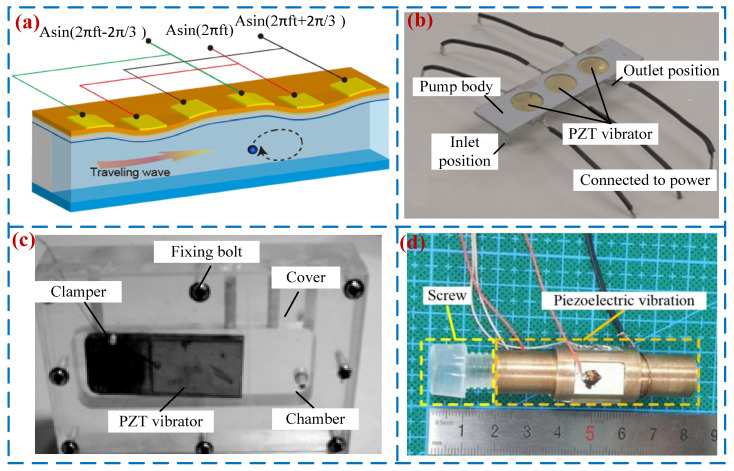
Dynamic actuation VLPPs: (a) an ultrasonic by Ogawa et al. [42], (b) a peristaltic by Zhang et al. [43], (c) a bionic by Huang et al. [44], and (d) a screw by Yin et al. [45].
